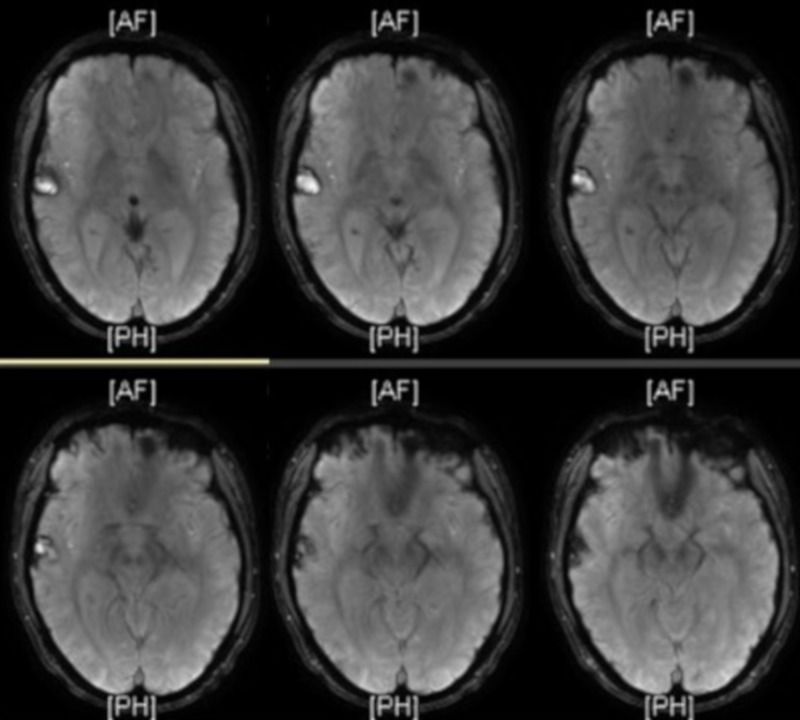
T1-weighted brain MRI revealed a persistent dominant focus in the right temporal region that was well demarcated and stable in size. It showed resolving white matter edema and improving post-traumatic foci of altered signal intensity when compared with prior imaging.
